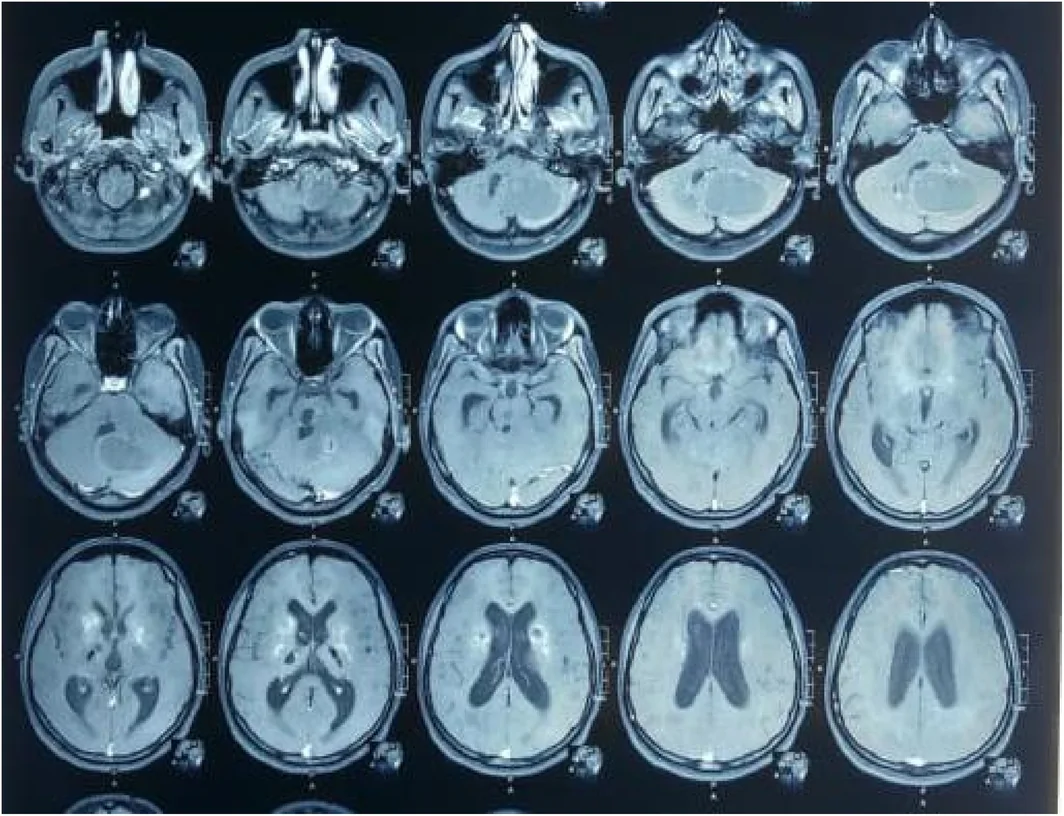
MRI Brain shows extensive bilateral basal ganglia and cerebellar calcification and cystic mass in left cerebellum compressing fourth ventricle producing obstructive hydrocephalus.
